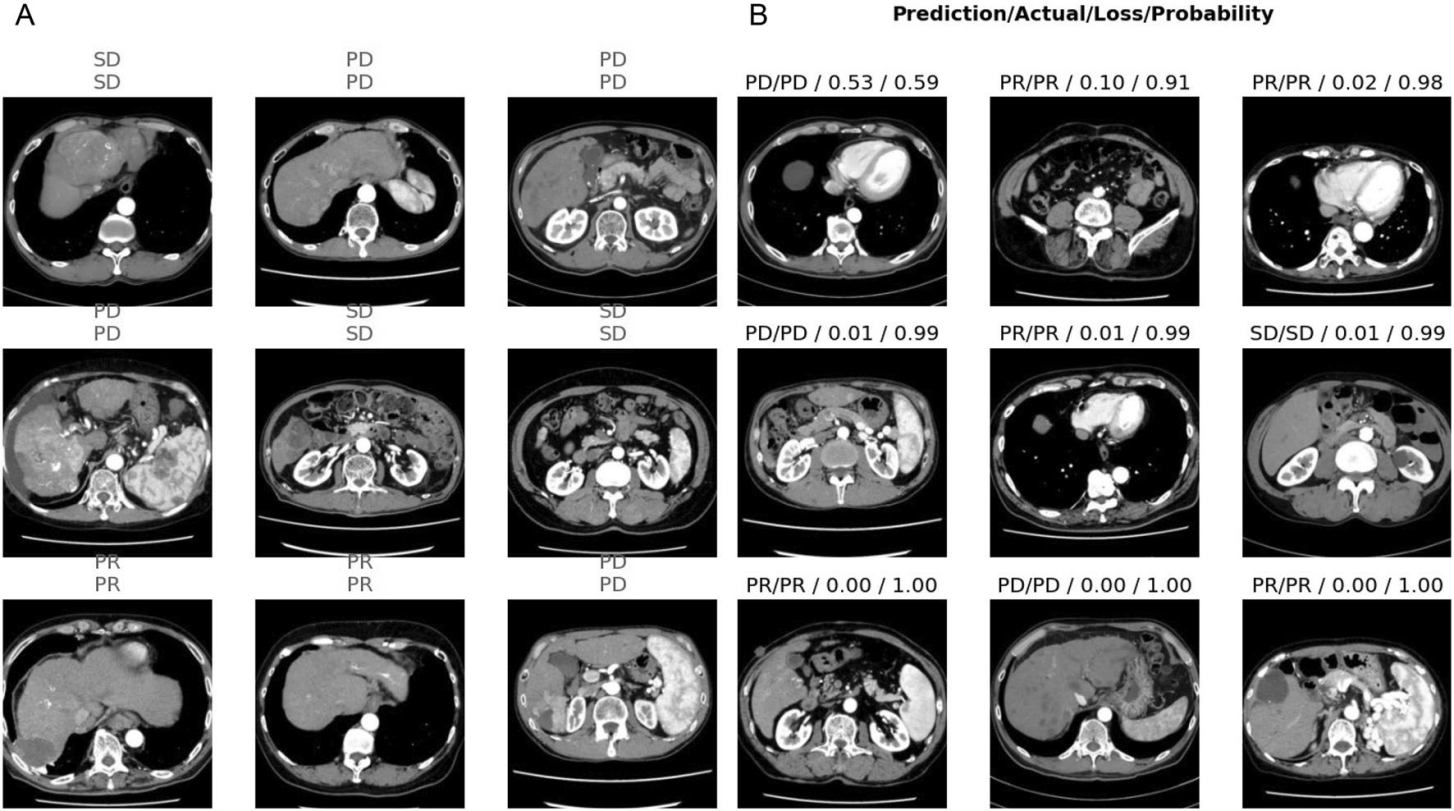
(A) Top label shows actual label second label shows the prediction model of each CT slices by ResNet-18, while (B) shows the computed loss and probability of each CT slices. CT, Computed tomography.
